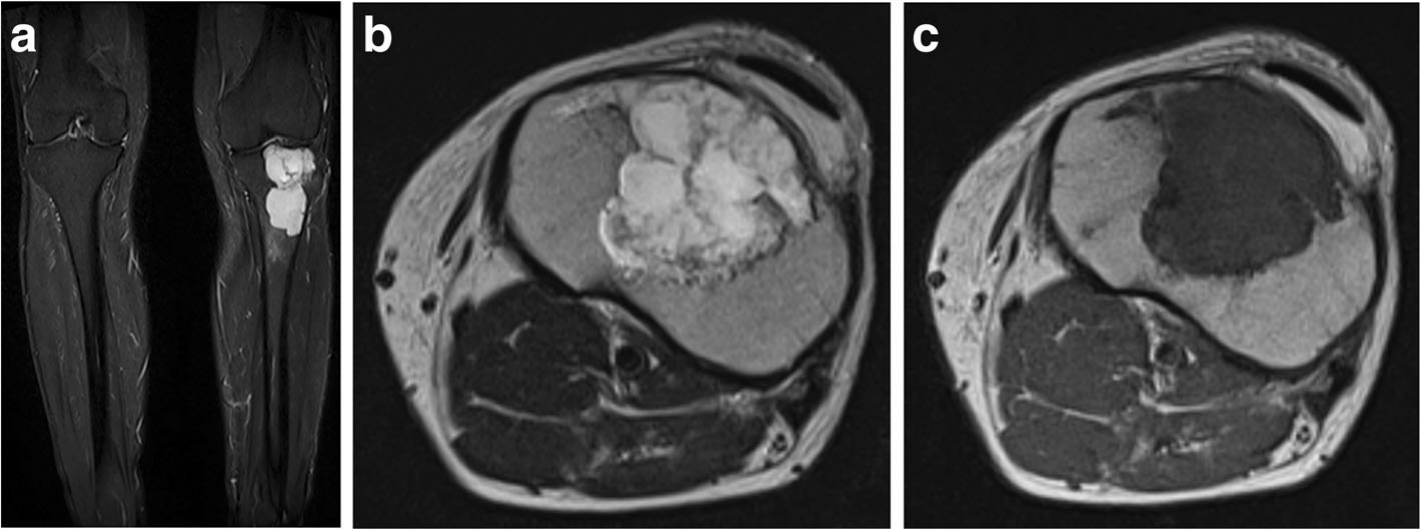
a–c MRI showed a multiloculated lesion with high T2, and Short-Tau Inversion Recovery (STIR) signals. The lesion is predominately a low T1 with mildly hyperintense patchy areas, which suggested intralesional haemorrhage. No fluid/fluid levels
